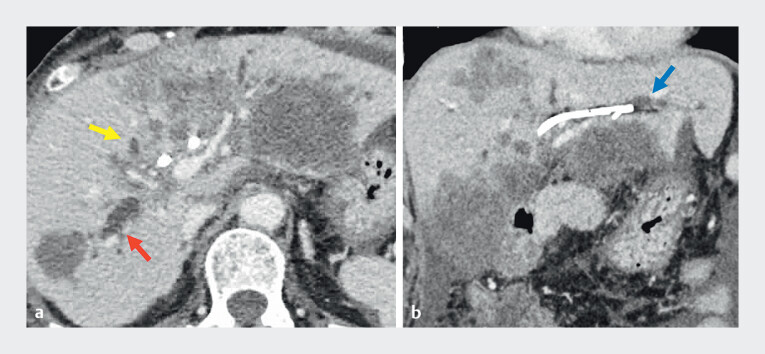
Contrast-enhanced CT showing progression of liver metastases with intrahepatic bile duct dilatation (yellow arrow, right anterior branch; red arrow, right posterior branch; blue arrow, left hepatic duct).
